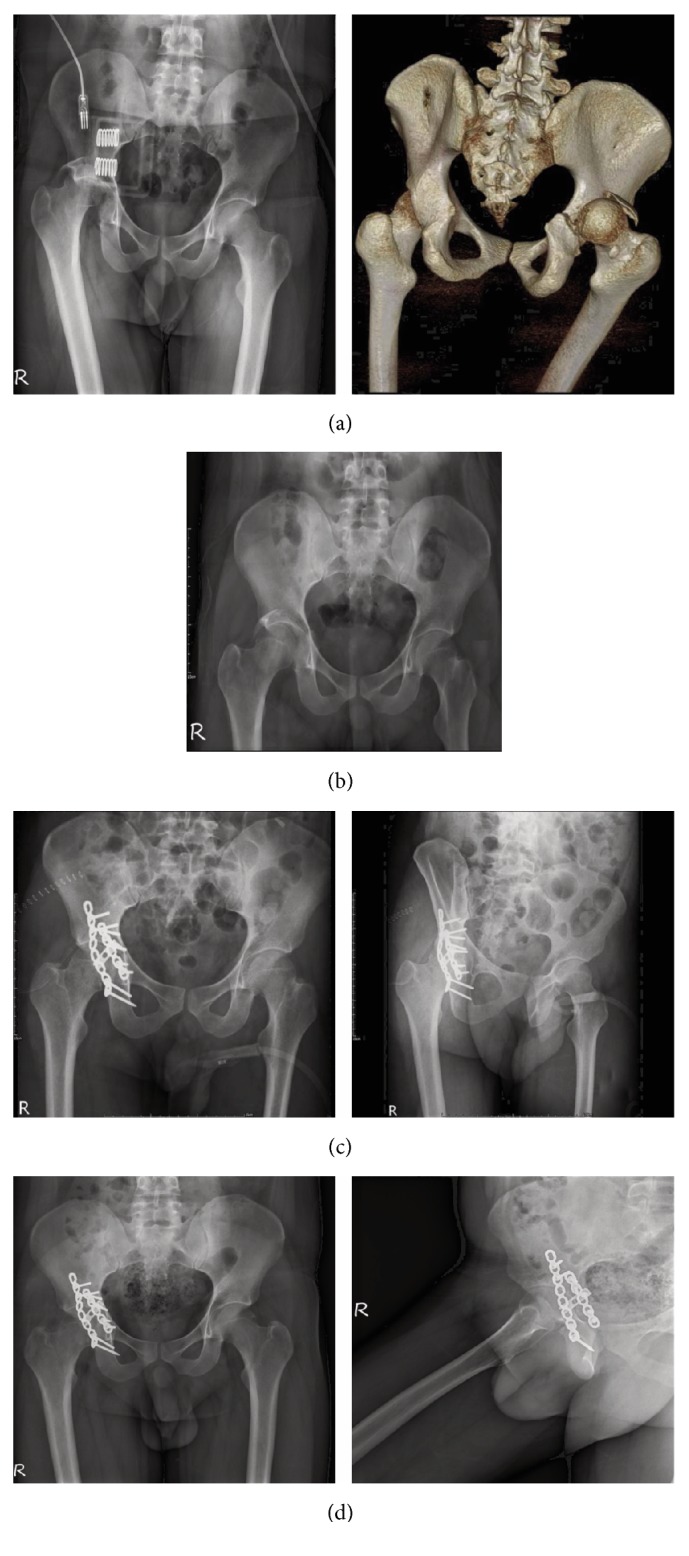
Images of posterior hip dislocation combined with transverse-type and posterior wall fracture of the right acetabulum in a 23-year-old man. (a) The posteriorly dislocated hip with acetabular fracture is shown by X-ray and 3D computed tomography images. (b) Status after closed reduction of the right hip with application of skeletal traction. (c) Open reduction via the Kocher-Langenbeck approach and internal fixation with three precontoured plates (anteroposterior and Judet views). (d) Postoperative follow-up radiographs at 4 months (hip anteroposterior and lateral views).
